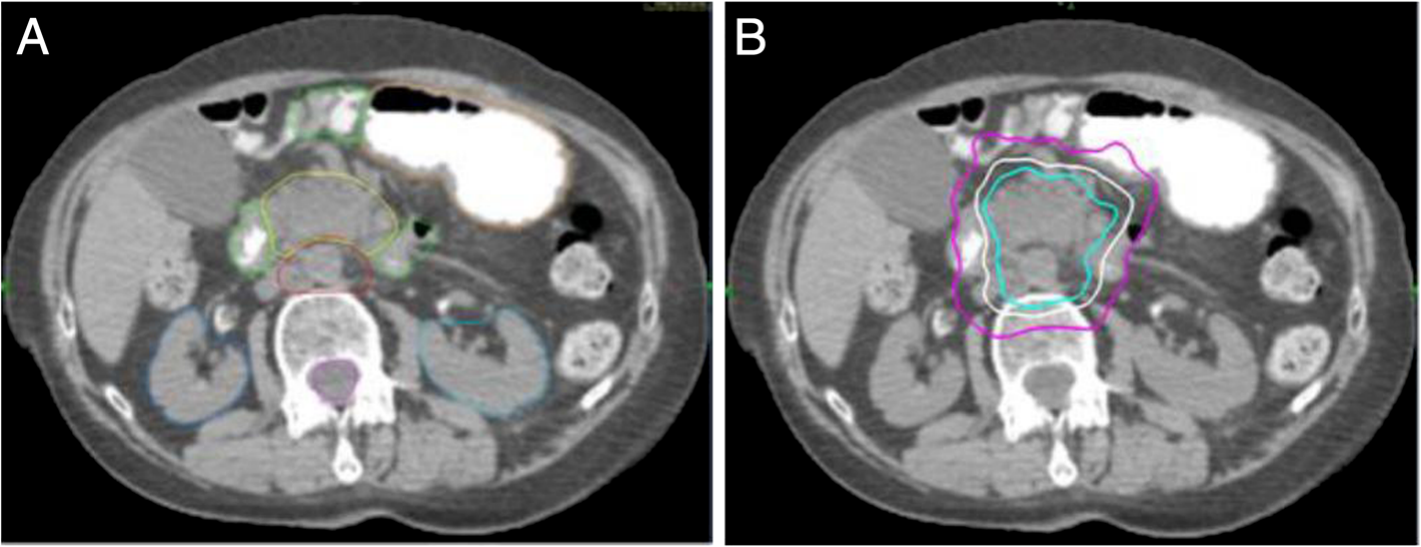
CyberKnife treatment planning for patient #2: A. Axial computed tomography images demonstrating the gross tumor volume (yellow), adjacent vascular volume (red), duodenum (green), stomach (orange), spinal cord (pink) and kidneys (blue). B. Isodose lines shown as follows: Blue 81% (prescription), white 70% and purple 50%.
